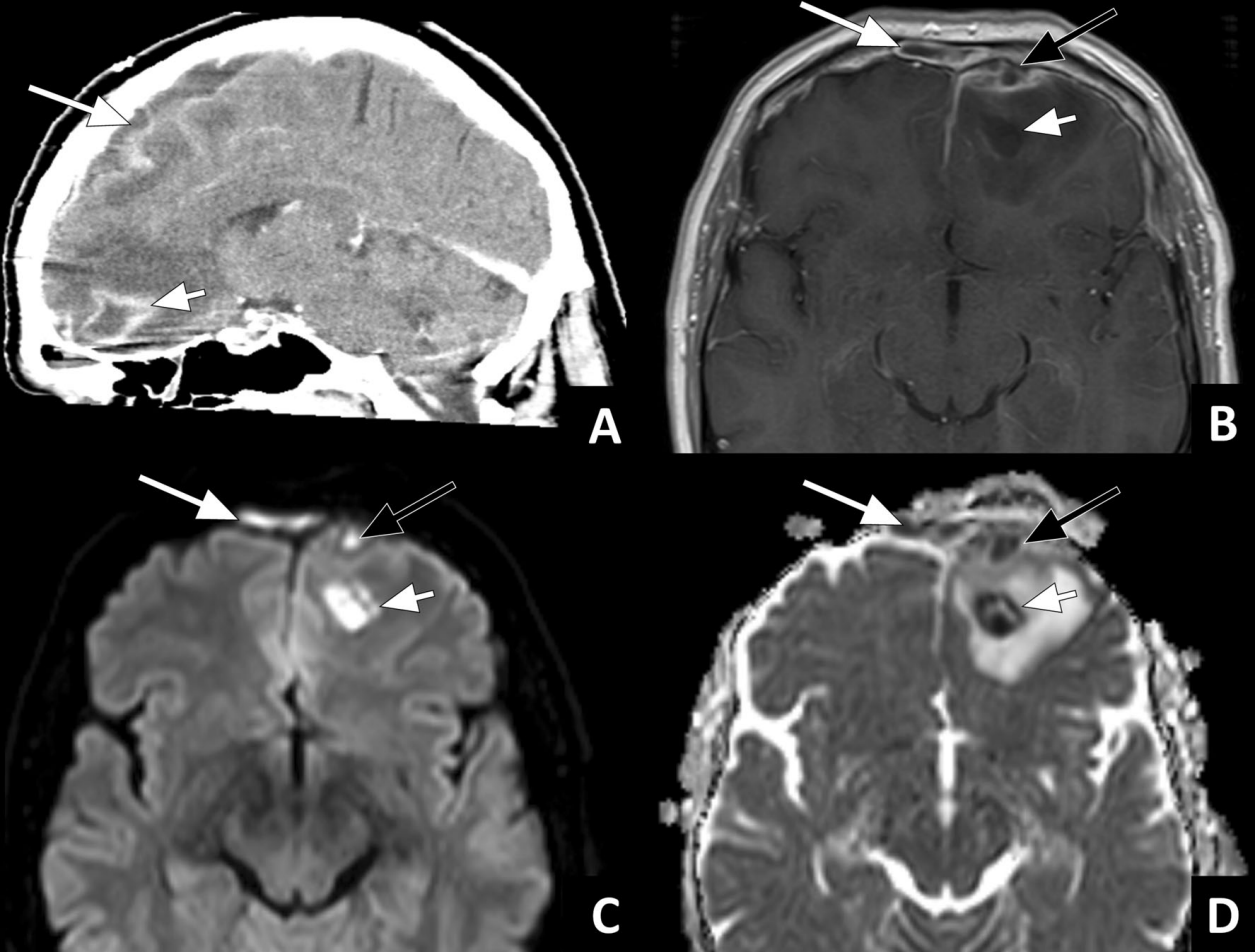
Brain abscess: a) Sagittal ceCT image showing an intra-axial fluid collection in the frontal lobe with rim enhancement (short arrow) and surrounding oedema. In addition, a subdural collection with thickening and enhancement of the meninges is present (long arrow). b) Axial T1w ceMRI, c) DWI b1000, and d) ADC map in the same patient showing a fluid collection in the frontal sinus with mucosal enhancement and restricted diffusion (long white arrow), a subdural fluid collection with thickened, enhancing meninges, and restricted diffusion (black arrow), and a fluid collection with rim enhancement in the left frontal lobe with restricted diffusion (short white arrow) and surrounding oedema. The findings are indicative of frontal sinusitis with pus formation, subdural empyema and brain abscess
